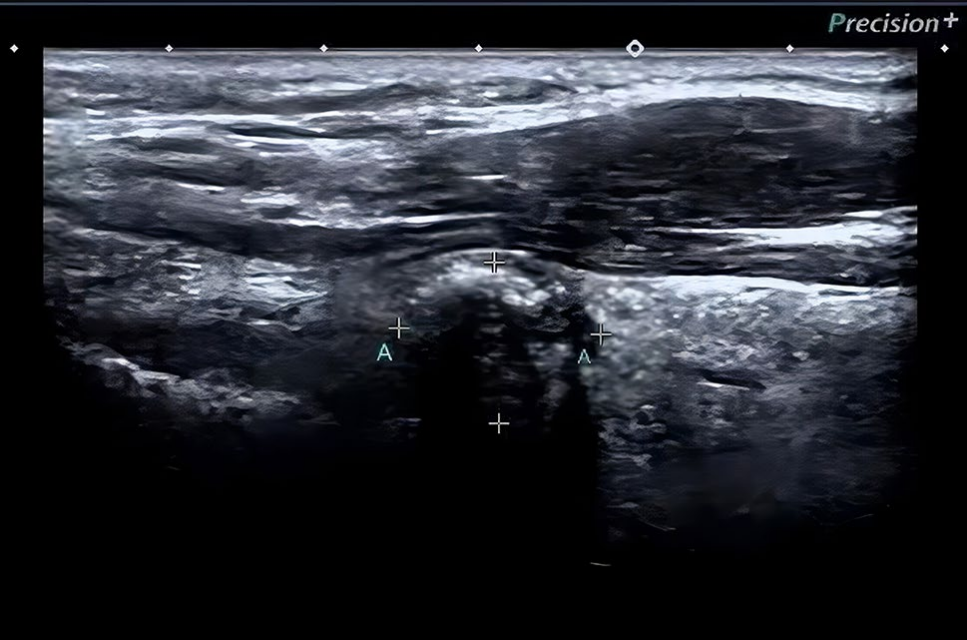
Ultrasound showed a 1.3 × 1.0 cm hypoechoic nodule with a faint border in the upper pole of the right lobe of the thyroid gland. (A: Thyroid mass)
